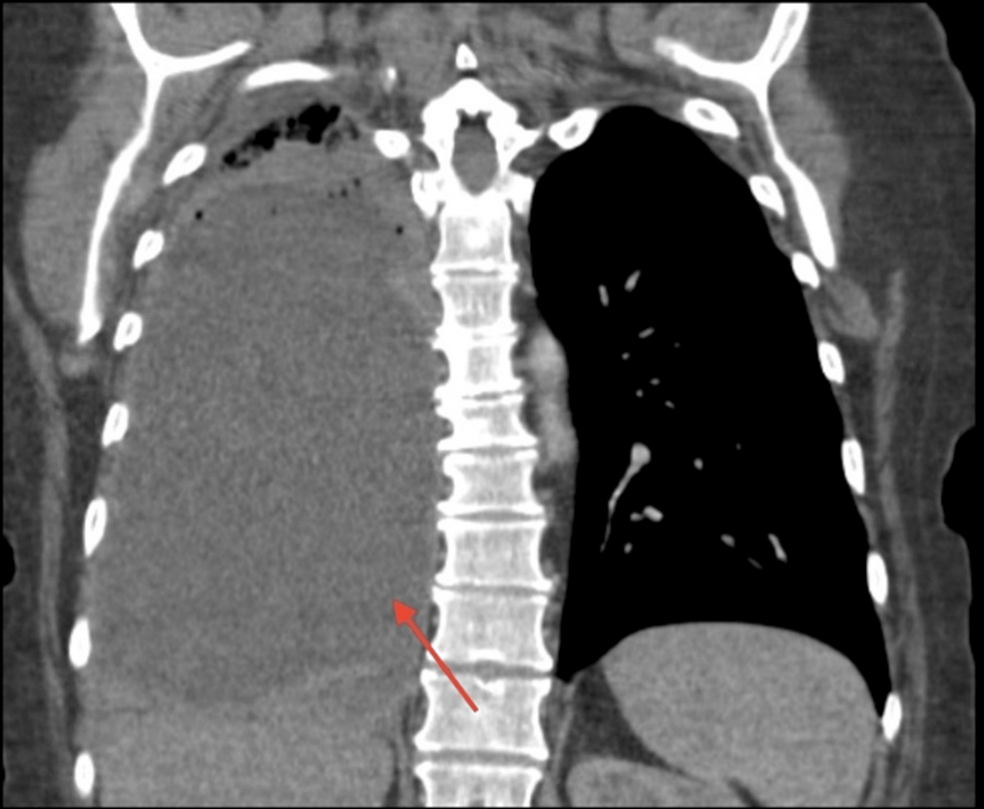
Outpatient CT of the Chest Red arrow indicating large right-sided large fluid collection. CT: computed tomography
